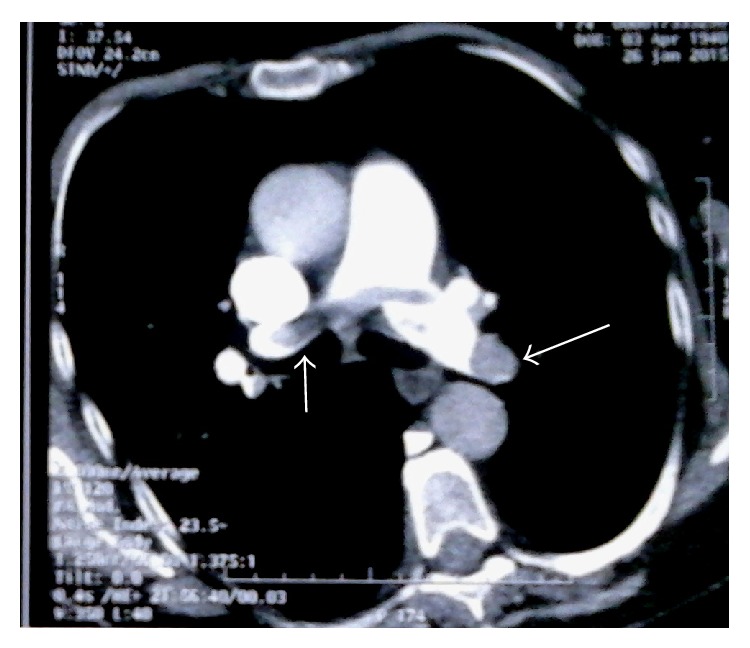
Computed tomography of the chest showing bilateral proximal pulmonary emboli (white arrows).
